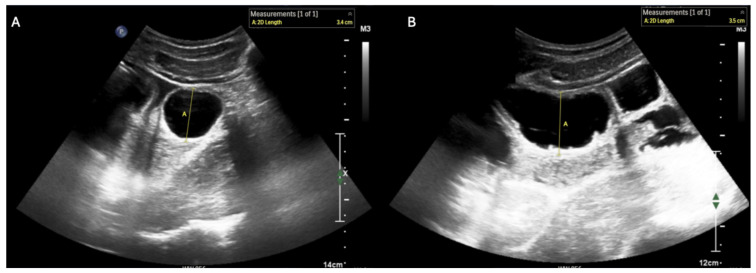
Short- (A) and long-axis (B) views of small bowel ileus, which has the following characteristics on ultrasound: (i) small bowel outer wall to outer wall diameter ≥ 2.75 cm (with 2.5–2.75 cm representing a borderline value) [8] and (ii) absent peristalsis [10]. Abnormal peristalsis is characterized either by (a) a complete absence of motion of intra-luminal contents or (b) “to-and-fro motion” of the bowel contents with a minimal decrease in size of the small bowel lumen (see Supplementary Video S3 for examples of these dynamic findings) [11].
